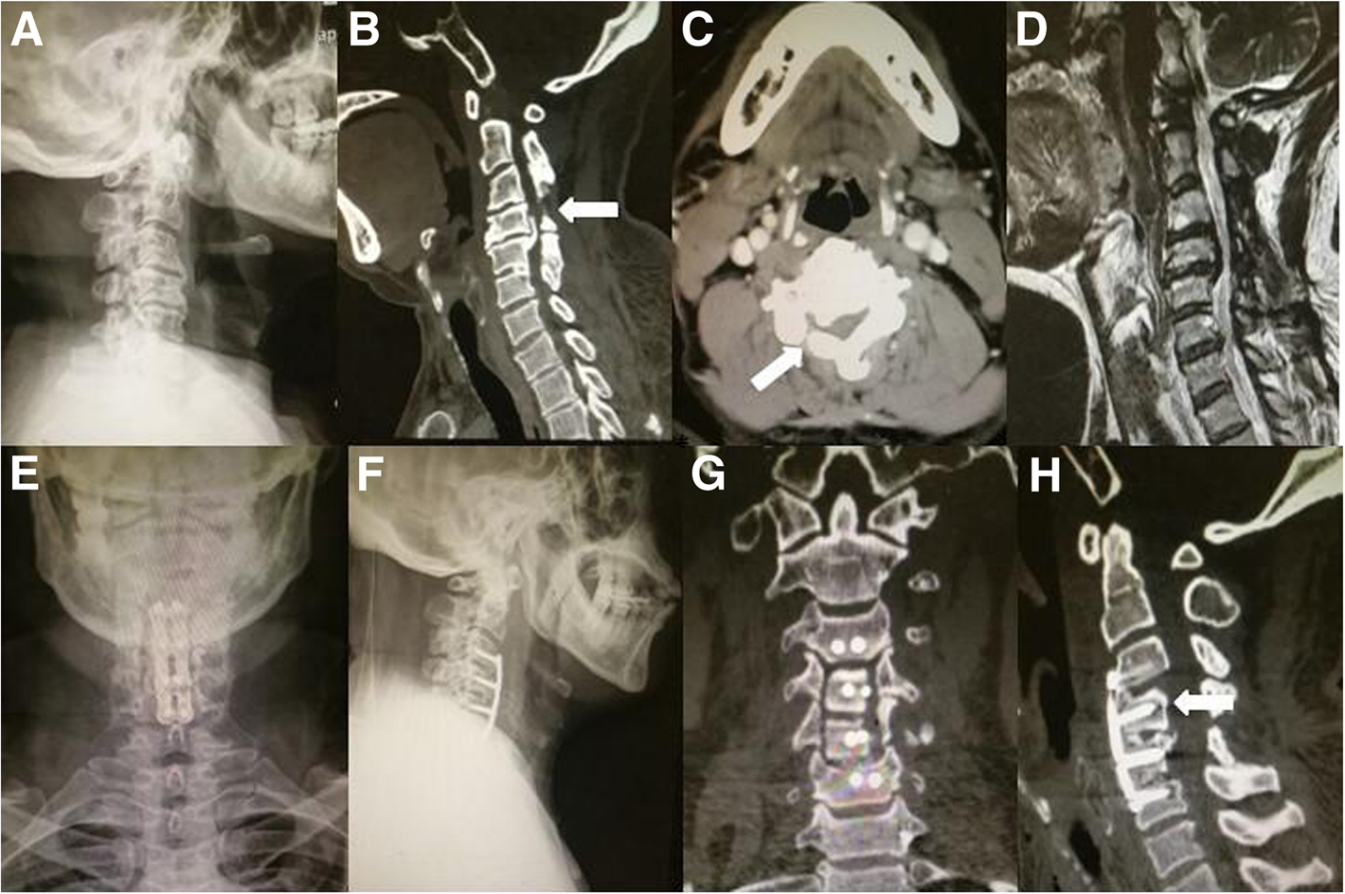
A revision case of a 59-year-old man 5 years after initial posterior decompression surgery. a The lateral image showed that cervical kyphosis occurred after the initial posterior surgery. b, c The CT scan showed that there was only a window decompressing without fixation in the initial posterior surgery (arrows). d The MRI showed that the cervical spinal cord of C4–5 was compressed by the OPLL. e, f The postoperative anterior–posterior and lateral images showed good device positioning and persistent poor cervical lordosis. g, h The postoperative CT scan showed that the bilateral troughs were created along the widest edge of the OPLL, and we hoisted the VOC by the screws (arrows). We usually used a 2-mm high-speed cutting burr and 1-mm Kerrison rongeurs to remove the posterior vertebral wall on the bottom of the troughs
